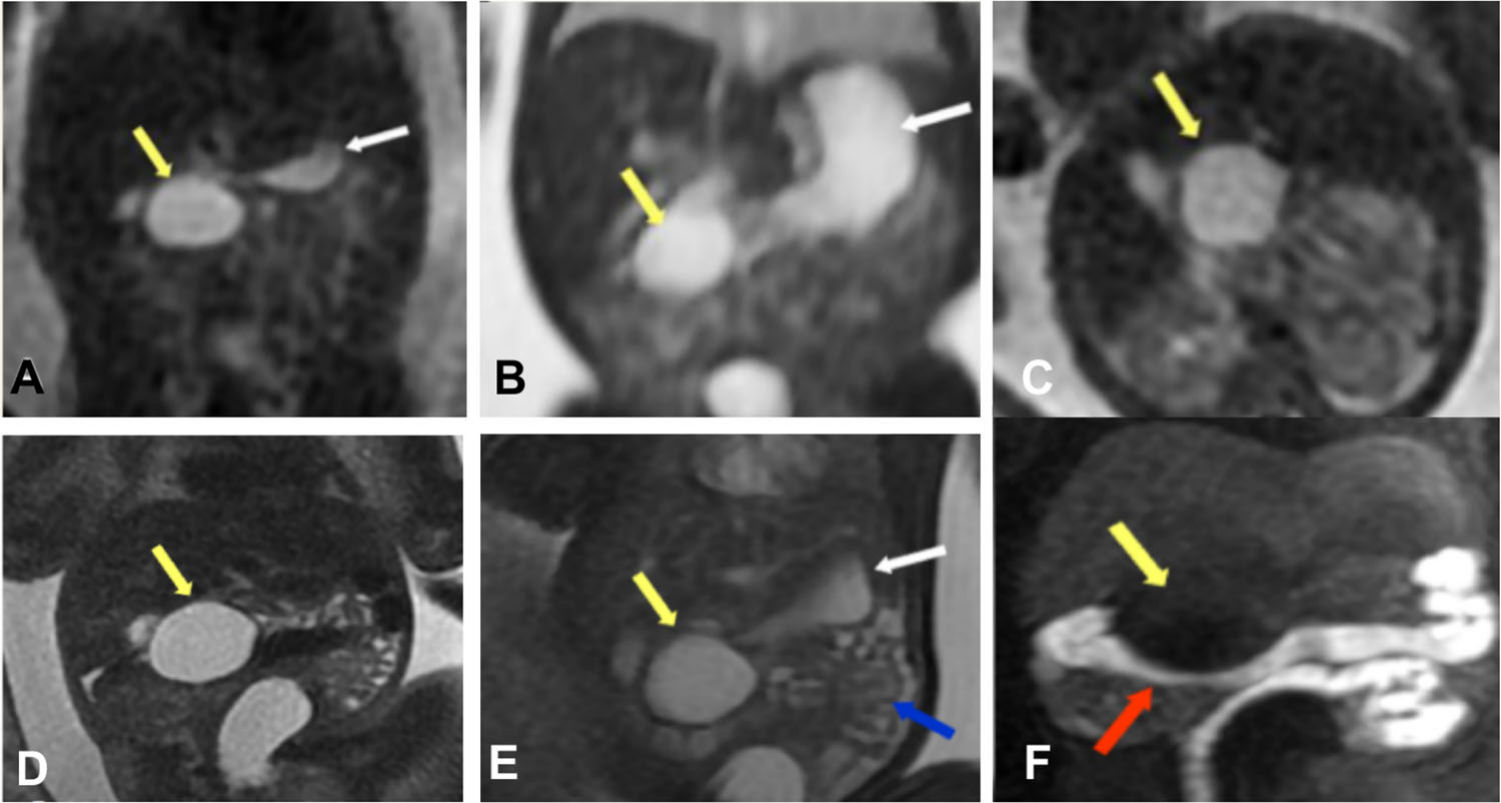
Intestinal Duplication Cyst. Fetal ultrasound showed a probable duodenal duplication cyst at a gestational age of 22 weeks and 1 day. A Coronal Single Shot FSE T2, (B) coronal FIESTA, and (C) axial SS FSE T2. Follow-up of the duodenal duplication cyst: (D) coronal SSFSE T2, (E) coronal FIESTA, and (F) coronal MIP 3D LAVA. The stomach is of normal size (white arrow), and the bowel loops are normal (blue arrow). The cyst indents the transverse colon (red arrows). A subhepatic cystic lesion is located anterior and medial to the second portion of the duodenum (yellow arrows). No connection with the stomach or the biliary tract is identified. MRI confirmed the ultrasound diagnosis
